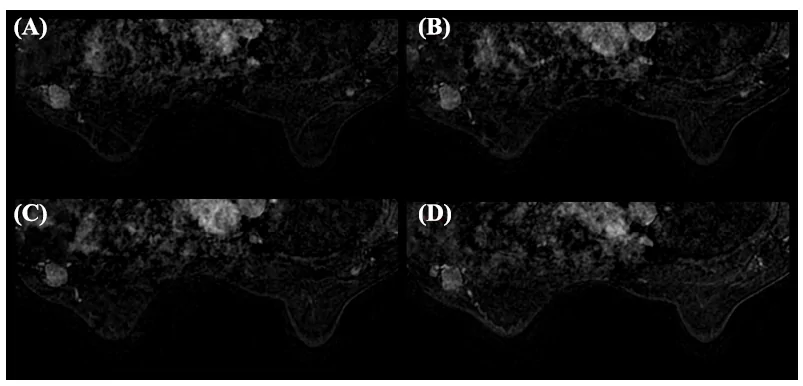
Representative axial T2-weighted fat-suppressed MR images showing morphological characteristics of axillary lymph nodes across four examples. (A) Non-metastatic lymph node with preserved fatty hilum and thin cortex (cortical thickness: 2.4 mm). (B) Metastatic lymph node with thickened cortex (cortical thickness: 7.4 mm) and loss of fatty hilum. (C) Another metastatic lymph node demonstrating cortical thickening (8.7mm). (D) Non-metastatic lymph node with normal appearance and thin cortex (2.9 mm).
